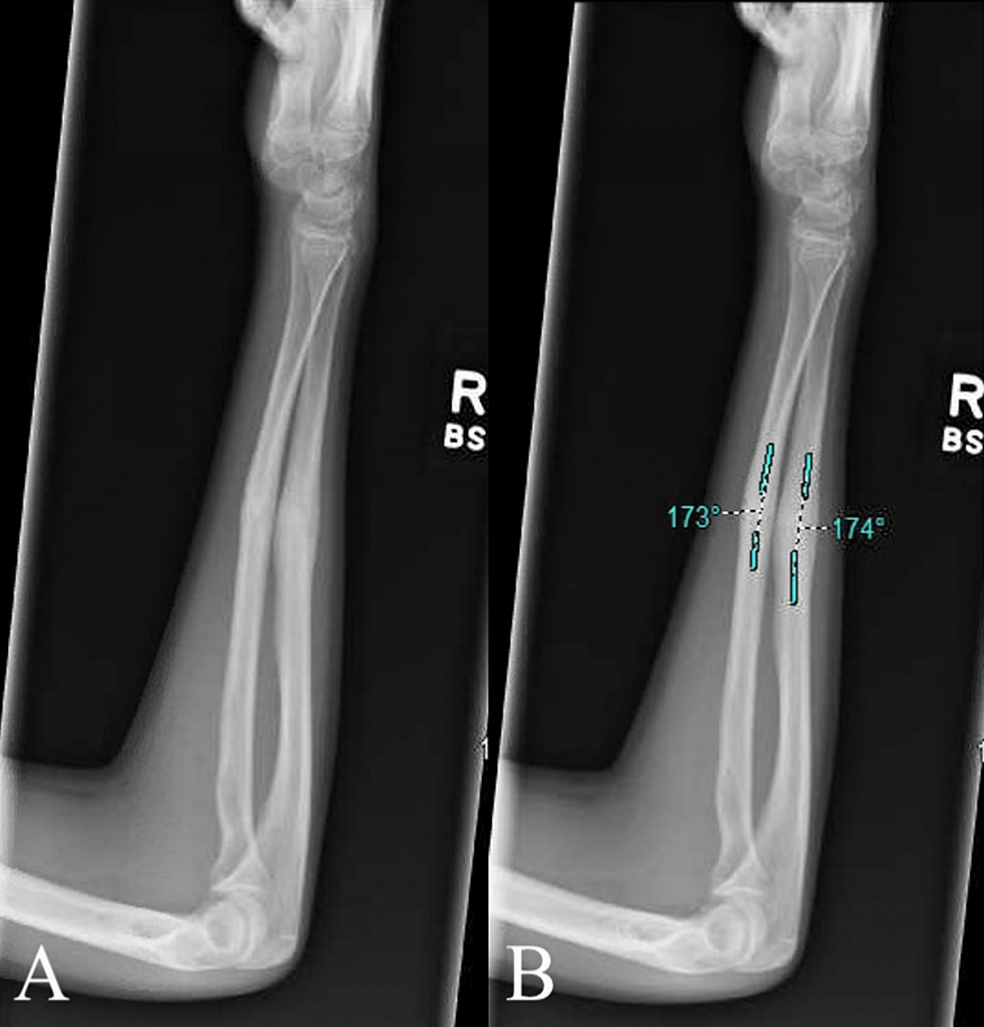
Post-reduction radiograph in lateral view with measurements unlabeled (A) and labeled (B). Blue markings measure the angle of deformity.
